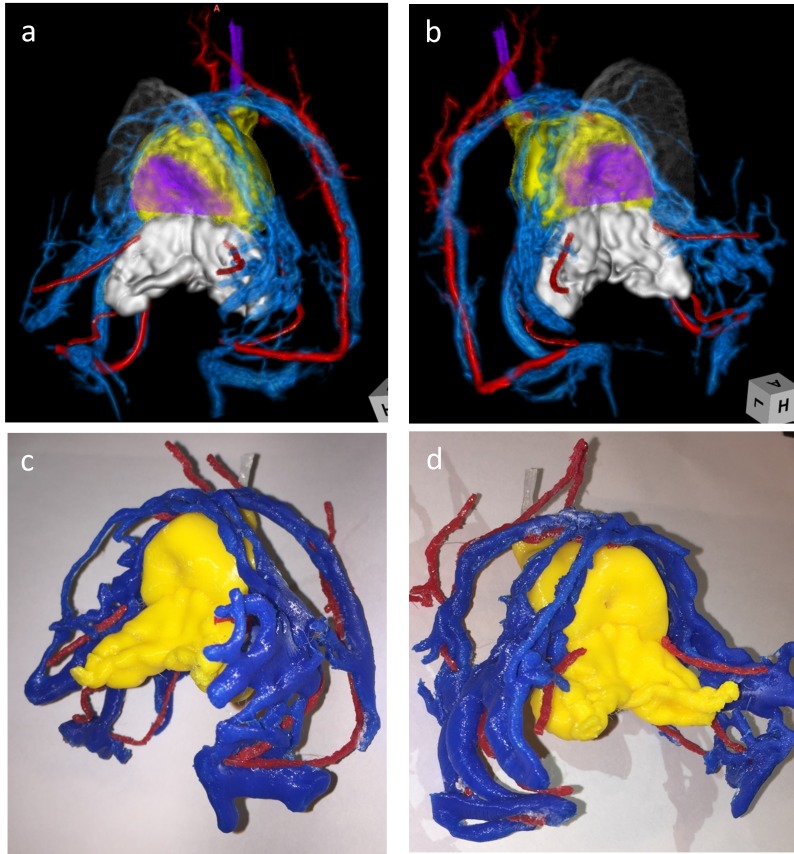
Three dimensional (3D) model of a typical case (Case 6) Volume rendered (VR) images (a, b) were created using T2 SPACE and true-FISP and contrast-enhanced T1-weighted VIBE. The soft tissues, such as prostate and seminal vesicles, were volume rendered using T2 SPACE. The representation of periprostatic blood vessels was generated using true-FISP and T1-weighted VIBE. The VR images were generated from standard triangulated language (STL) files constructed for 3D printing. Arteries in each image are represented in red and the veins in blue.
